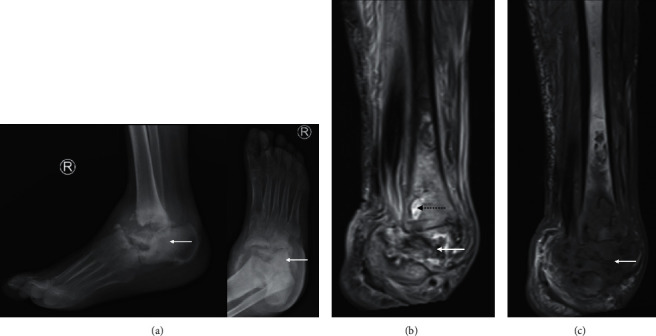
Lateral and oblique right foot radiographs (a) of a 47-year-old woman with type II diabetes and Charcot arthropathy involving the midfoot. Disorganised hindfoot with joint destruction and disorganisation (arrow). Note the absence of osteopenia that is typically observed in infection. Coronal STIR (b) and T1 (c) of a different patient with Charcot arthropathy with hindfoot destruction, hindfoot valgus, and dislocation. The contrast was not administered due to chronic renal failure. The talus is flattened and sclerotic (arrow). Note the fluid collection in the distal tibial shaft (dotted arrow) and the medial tibiotalar articulation. This patient had both neuropathic arthropathy complicated by chronic osteomyelitis with intraosseous abscess formation. At times, different conditions can preexist, and ultimately, tissue sampling may be required.
